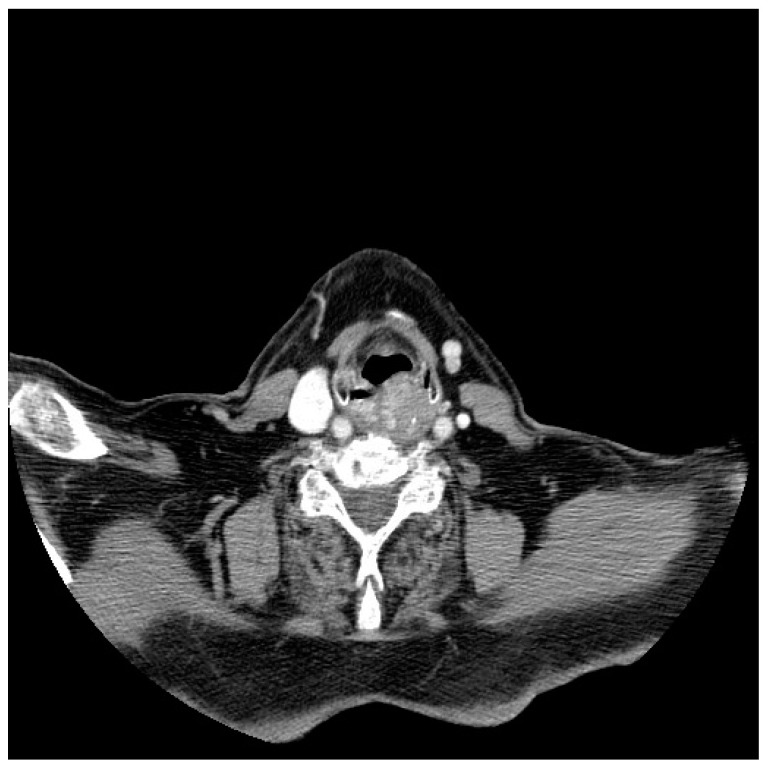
CT scan of a patient with a laryngeal well-differentiated liposarcoma.
